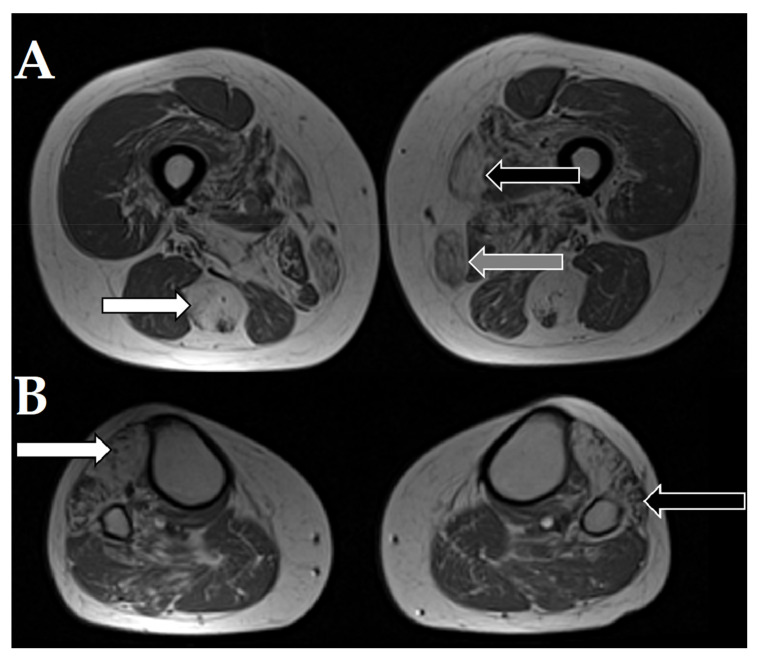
Magnetic resonance imaging of selected axial T1W images of muscles of the patient 1a with desminopathy. (A). White arrow points to severe fatty atrophy in the semitendinosus muscle. Black arrow points to the sartorius muscle. Grey arrow points to the gracilis muscle. (B). White arrow points to severe changes in the tibialis anterior muscle. Black arrow points to the affected peroneus muscles.
